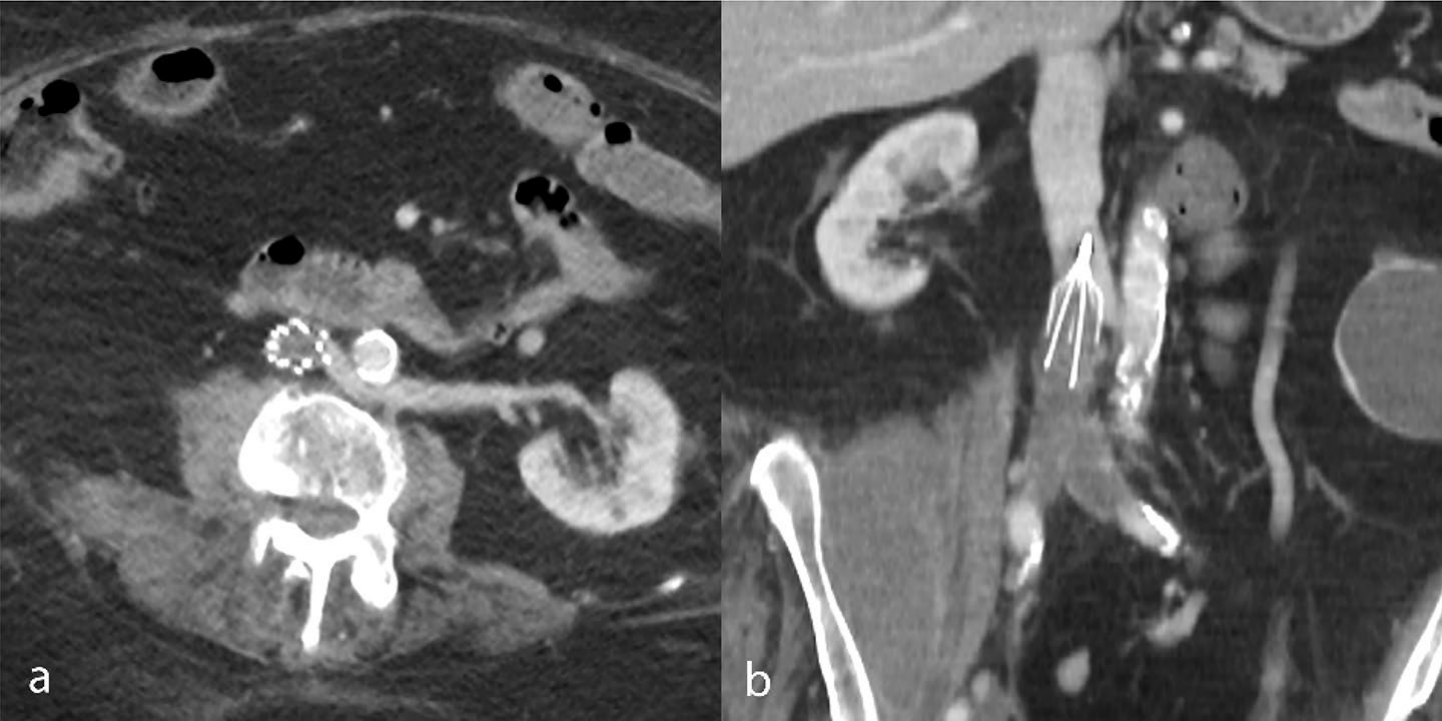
CT venography obtained one month after IVCF placement shows filter tilt and acute DVT of the iliac veins and infrarenal IVC on axial (a) and coronal reformatted image (b), leading to technical ineligibility for retrieval. *CT* computed tomography, *DVT* deep venous thrombosis, *IVC* inferior vena cava, *IVCF* inferior vena cava filter.
